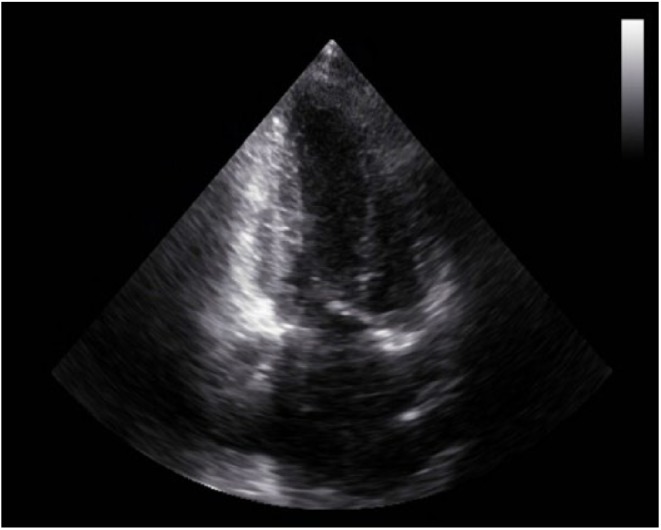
Baseline apical 2-chamber view of transthoracic echocardiogram 2 months ago showing normal basal to distal anterolateral, and inferoseptal walls of LV without any outpouching or aneurysm.
